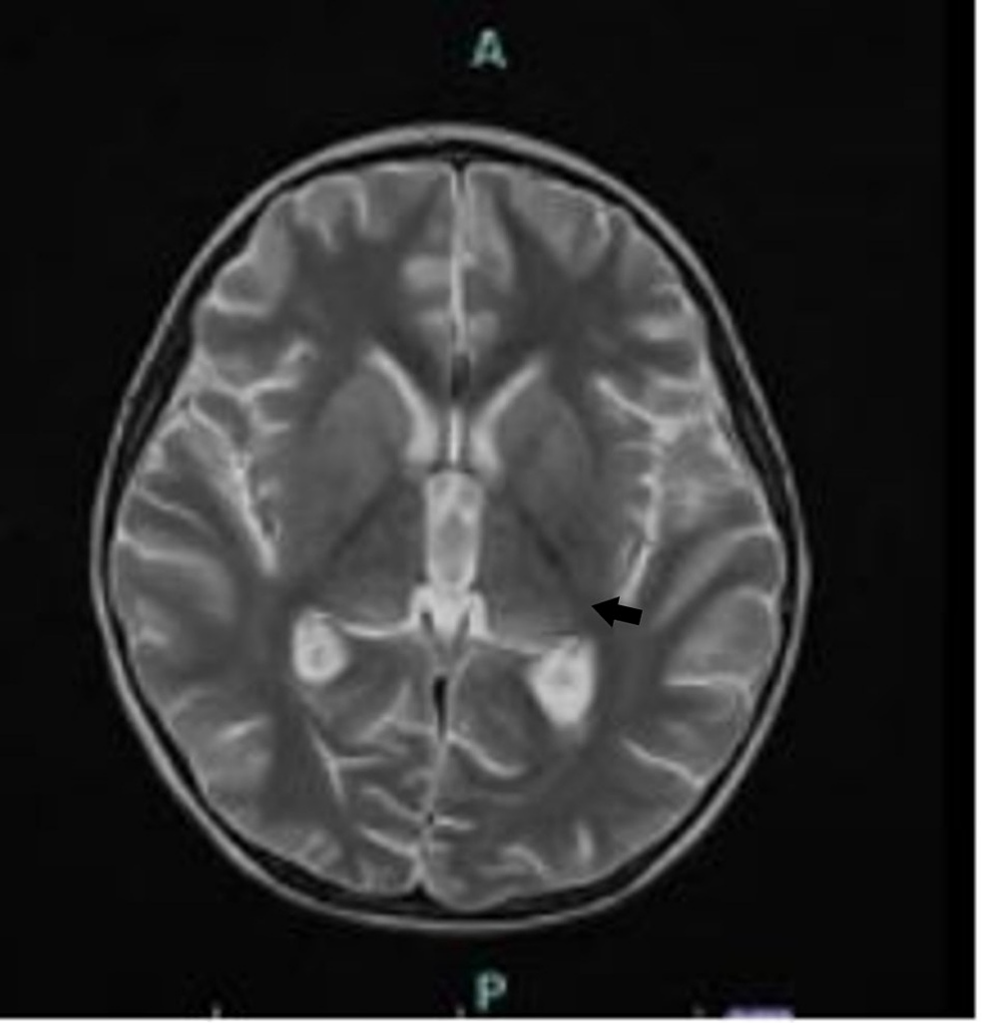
Axial T2 25 days later shows resolution of MRI changes, Normal appearance of the thalamus (arrow).
